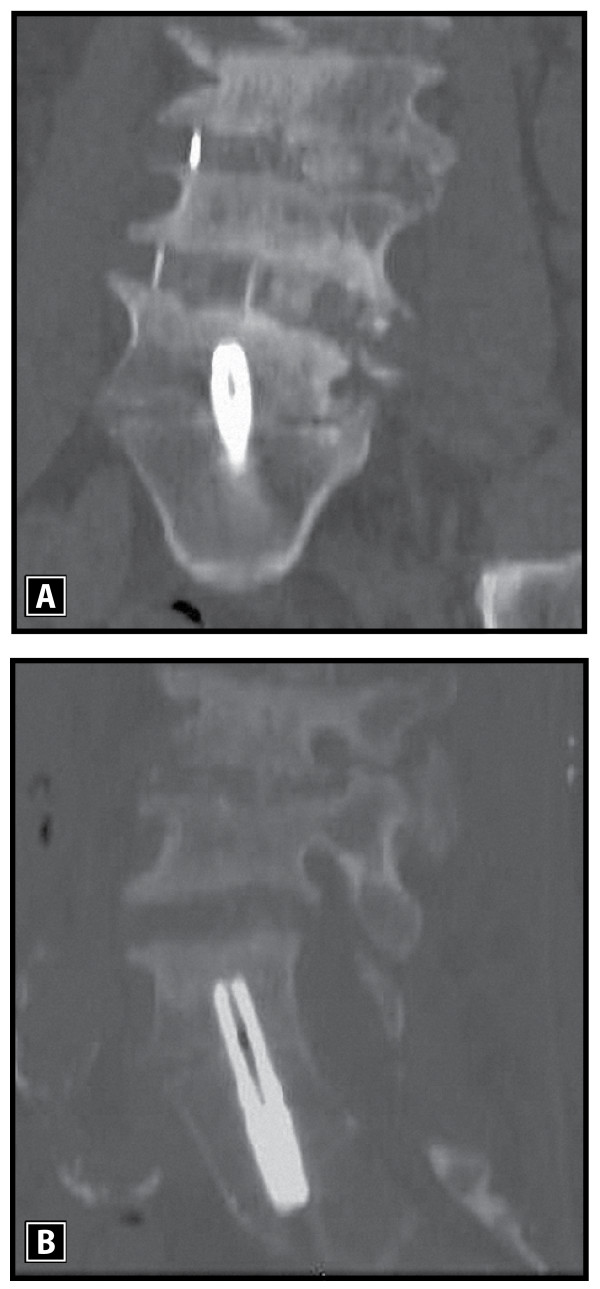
Coronal (A) and sagittal (B) computed tomography (CT) images demonstrating loss of L5-S1 disc space with recurrence of symptoms due to direct sacral impaction following a fall six weeks after the index surgery.
